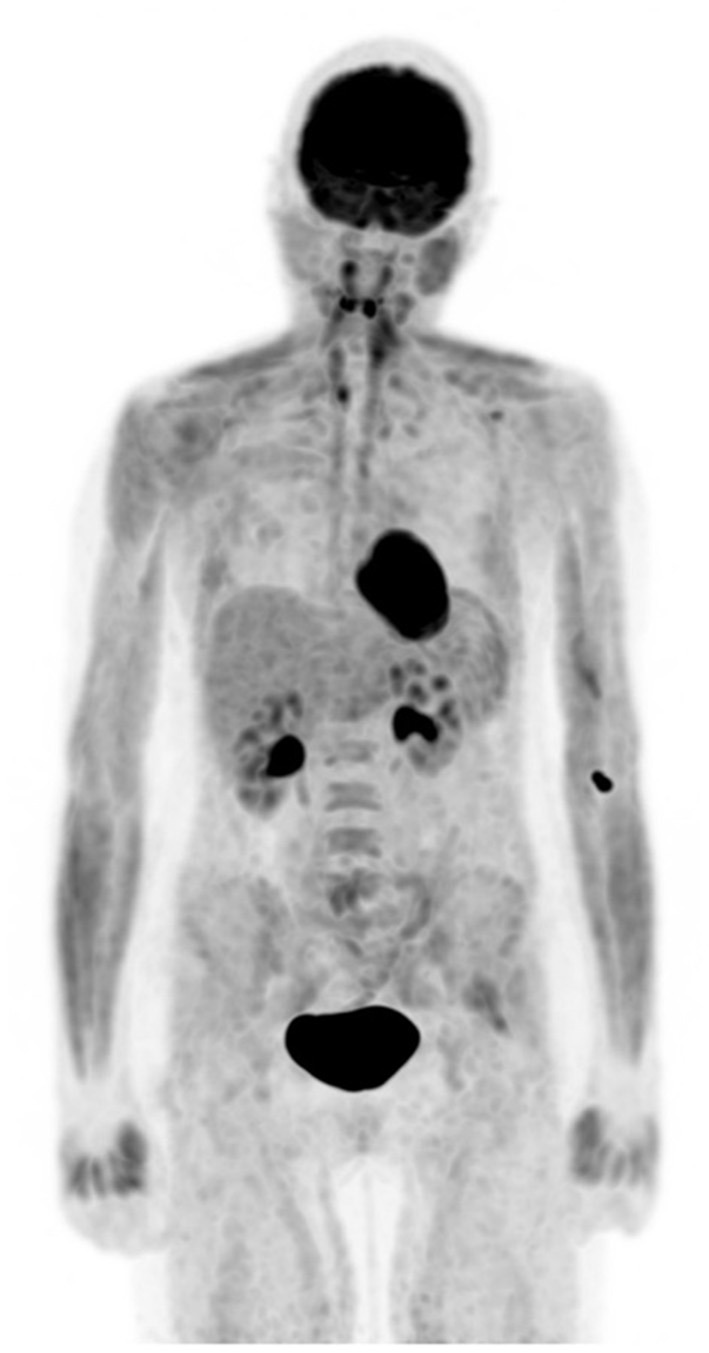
Altered biodistribution. FDG PET maximum intensity projection (MIP) image demonstrates diffuse increased muscular uptake in a patient who admits having a meal about an hour before coming for injection despite the knowledge of preparation for study.
